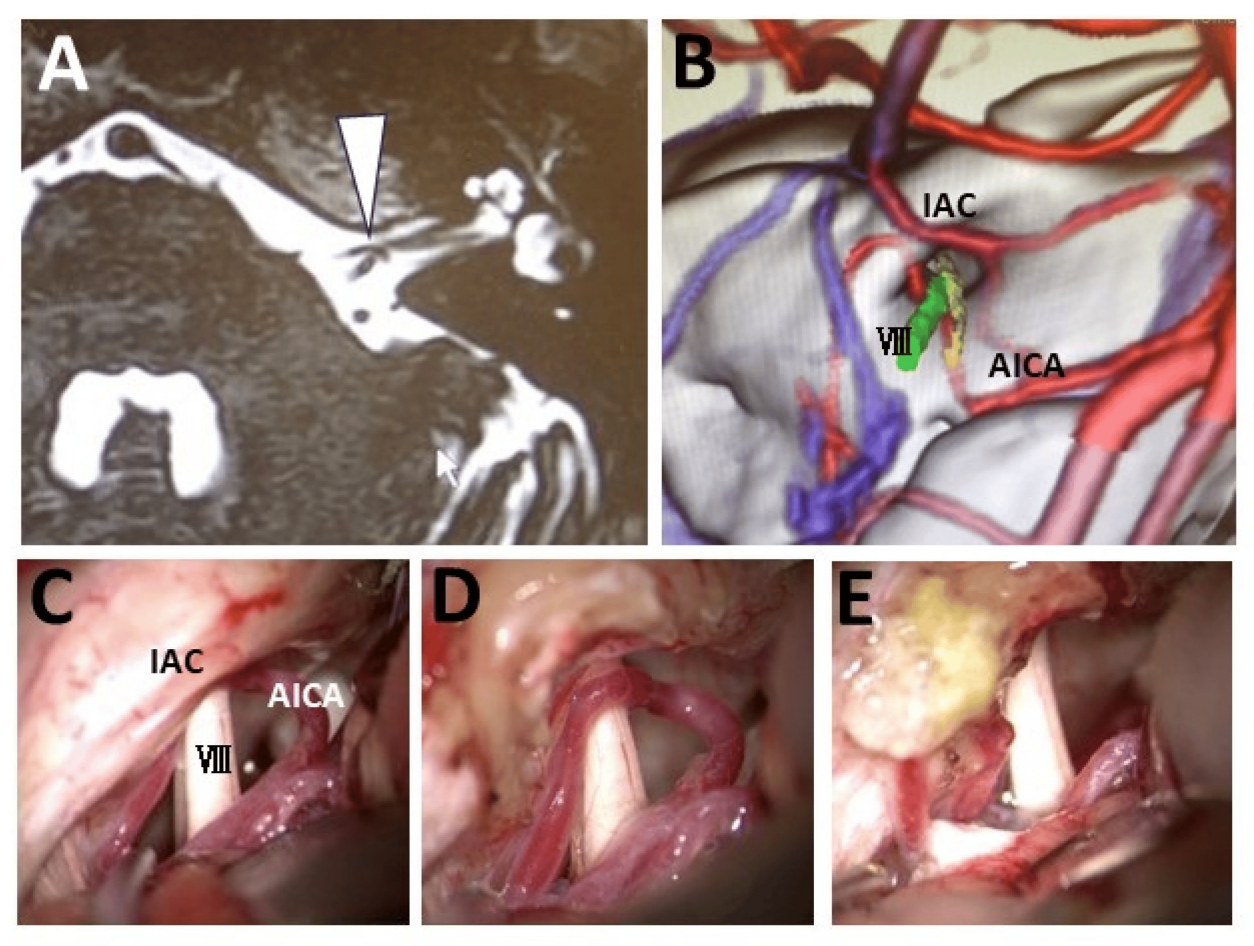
Preoperative imaging and intraoperative field images of Case 1 A: Preoperative head CISS MRI showing the left elongated AICA forming a loop within the IAC and compressing the vestibular nerve (VIII) at the internal acoustic meatus (white arrowhead). B: Fusion image of 3D CT angiography and head MRI showing the AICA compressing the vestibular nerve. C–E: Intraoperative findings confirming cranial nerve compression by the AICA after opening the internal acoustic meatus (C and D), and hence transposition of the AICA was performed (E) AICA: anterior inferior cerebellar artery; CISS: constructive interference in steady state; CT: computed tomography; IAC: internal acoustic canal; MRI: magnetic resonance imaging
